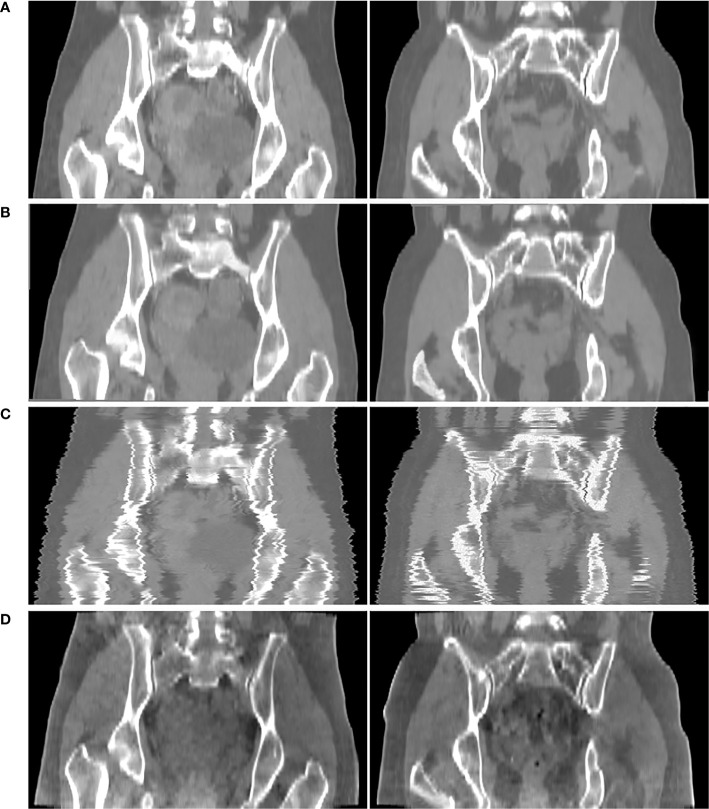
Comparison results of CT_gt_ and pseudo CT obtained based on 3D and 2D CycleGAN. (A) real CT images(CT_gt_). (B) pseudo-CT images obtained based on 3D CycleGAN. (C) pseudo-CT images obtained based on 2D CycleGAN. (D) CBCT images.
